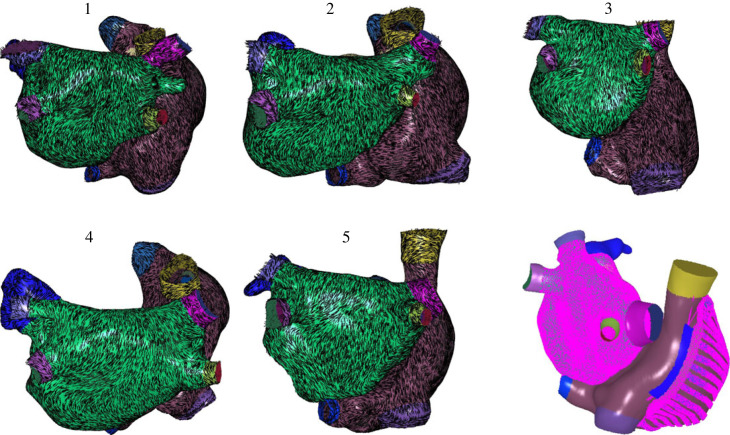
A cohort of five MRI models with fibres. The final panel shows the locations of line connections (in purple) that couple the different surfaces in the bilayer model representation.
